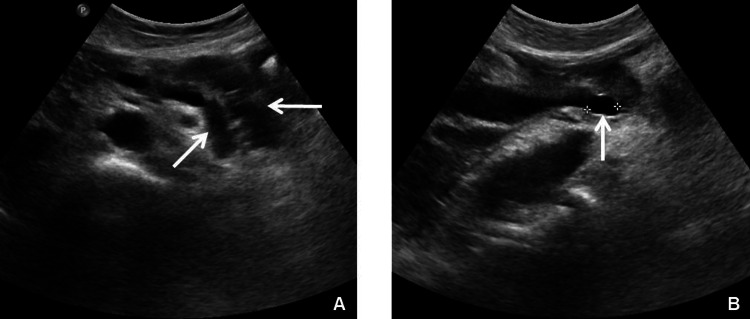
Abdominal ultrasound of February 2017 (A) Horizontal arrow indicates reduced dimensions of the pancreas. Oblique arrow indicates dilatation of Wirsung duct.
